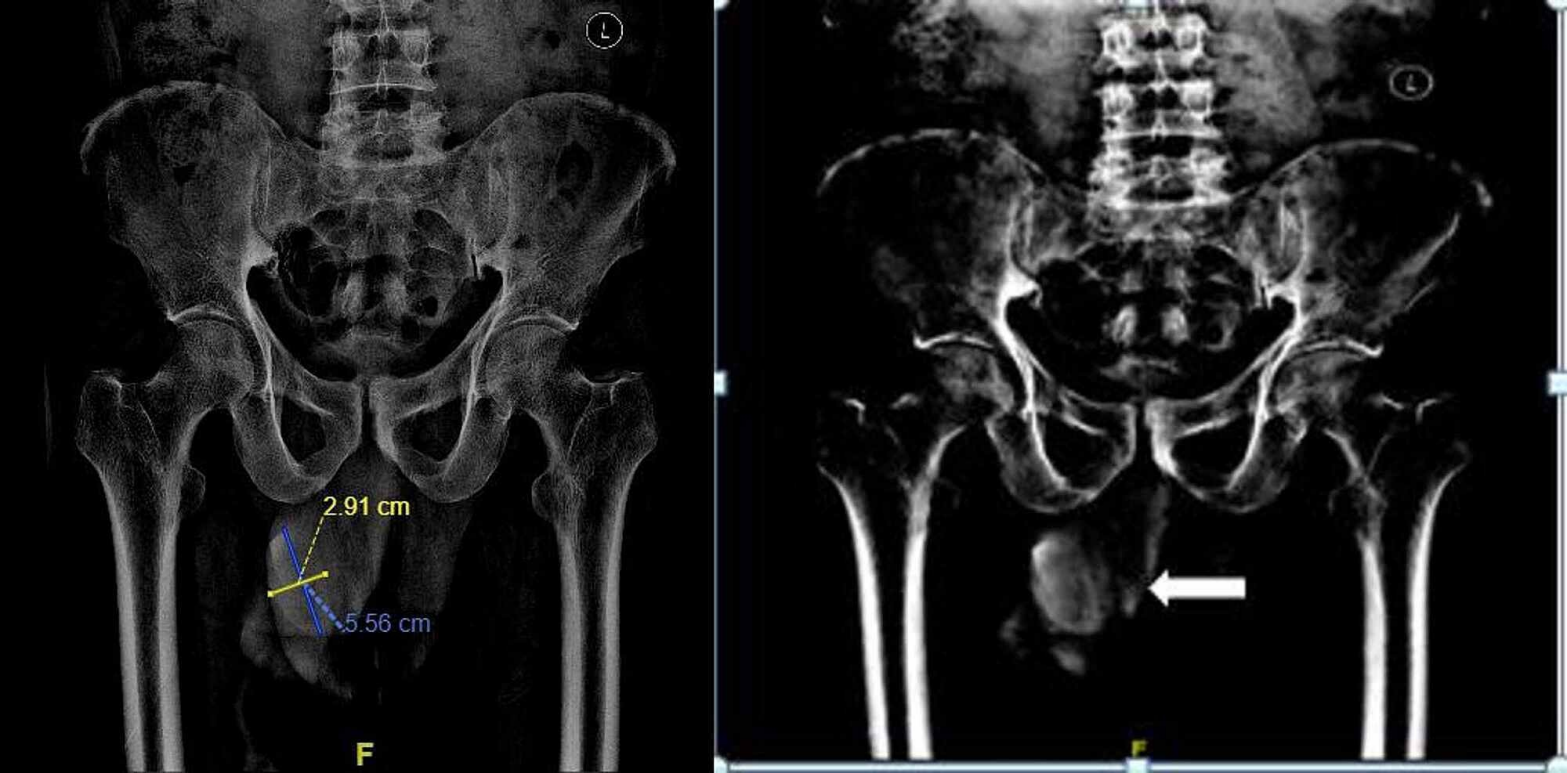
X-Ray pelvis demonstrating the calculus (shown by arrow) over the glans
